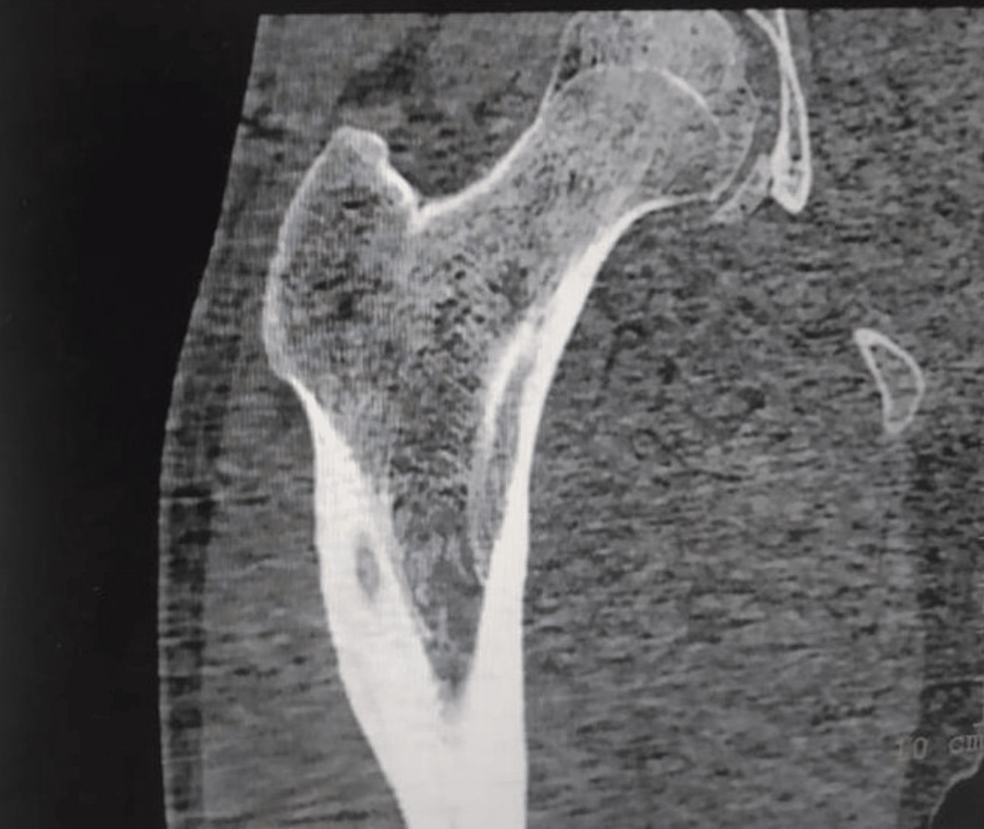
Coronal section of multidetector computed tomography scan of the proximal femur showing osteoid osteoma
